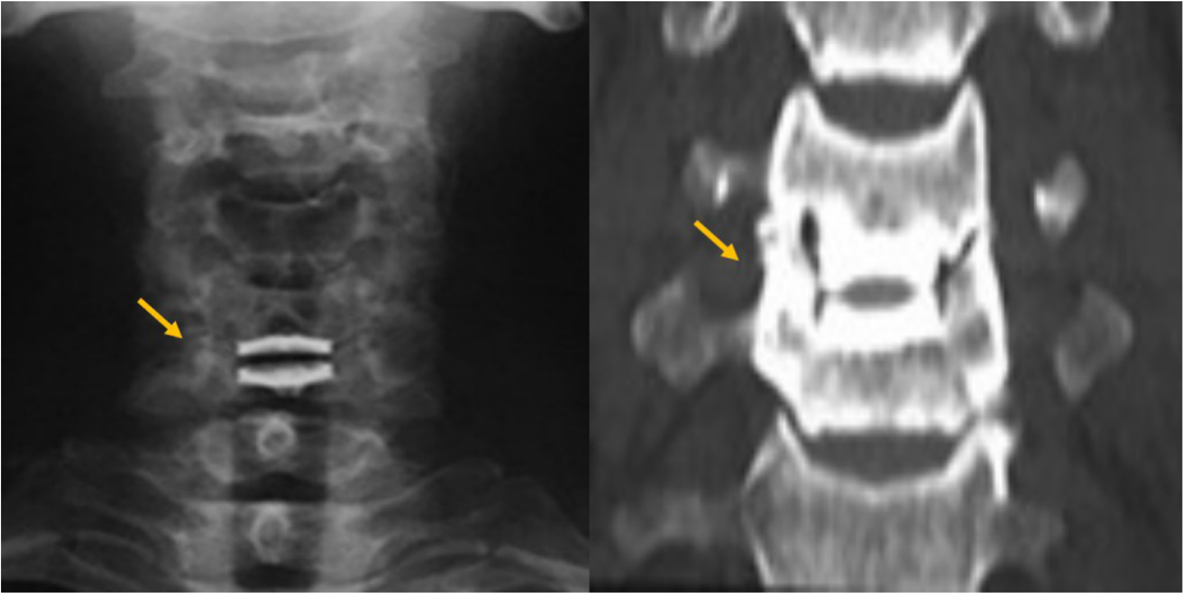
Uncovertebral joint ossification is observed in anteroposterior views from computed tomography and X-ray images
